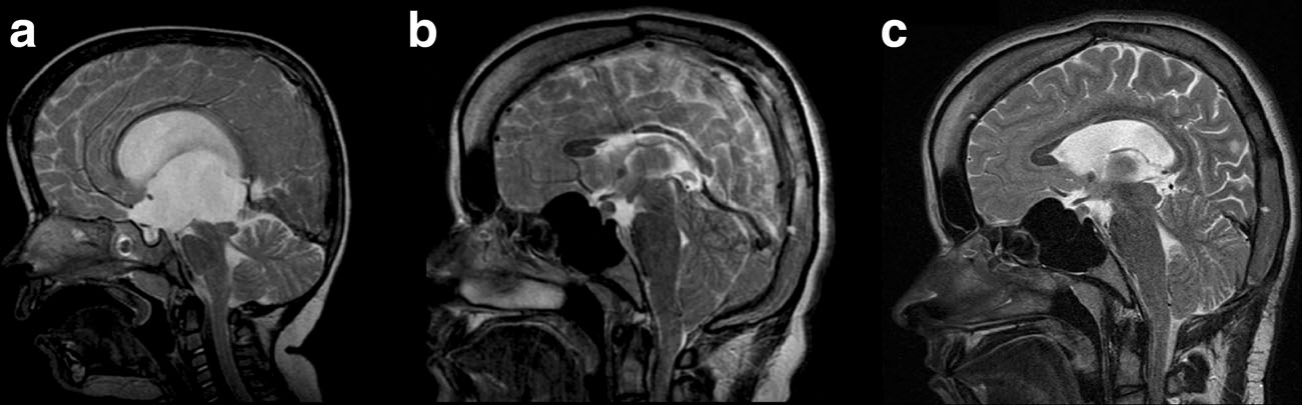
MRI scans of a 3-year-old girl with hydrocephalus due to aqueductal stenosis (a). She was treated with a third ventriculostomy and a ventriculoperitoneal shunt. Several years later, she developed an acquired Chiari I malformation with cranial vault thickening (b). After augmentation of the posterior fossa by thinning the occipital planum and decompression of C0 and a C1 laminectomy with dural patch grafting, the postoperative MRI scan shows an adequate decompression (c)
